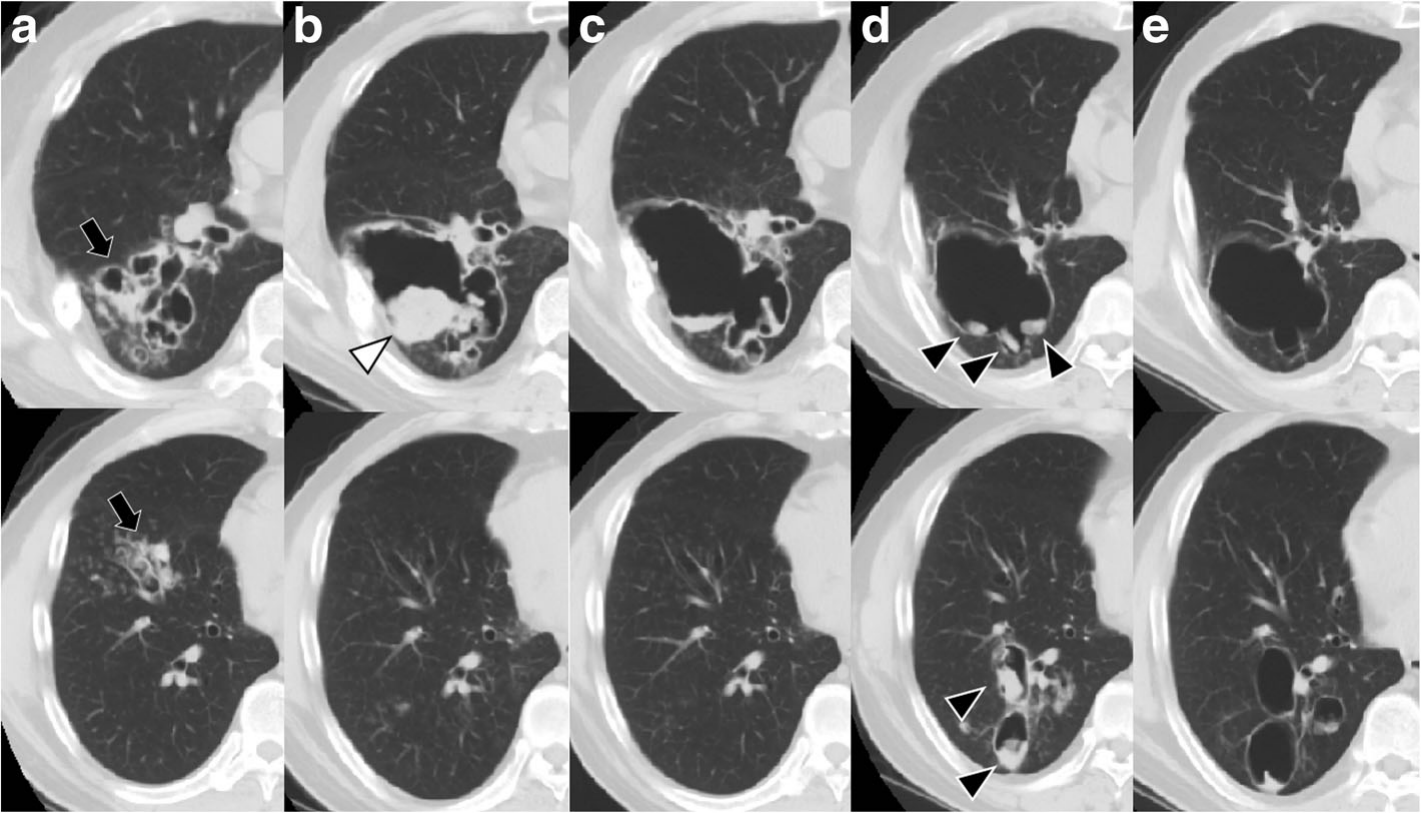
Chest computed tomography (CT) at the time of relapse (a), 2 months (b), 3 months (c), 22 months (d), and 6 years after the relapse (e). (a) Recurrence of bronchiectasis and cavities with mucoid impaction (black arrow) in the right lower lobe. (b) A cavitary lesion with a fungus ball (white arrowhead) was observed in the right lower lobe. (c) Improvement seen on CT after additional treatment with voriconazole. (d) CT scan showing advancement of fungus balls inside the large cavity in the right lower lobe (black arrowhead). (e) No fungus balls seen on CT 6 years after the relapse
